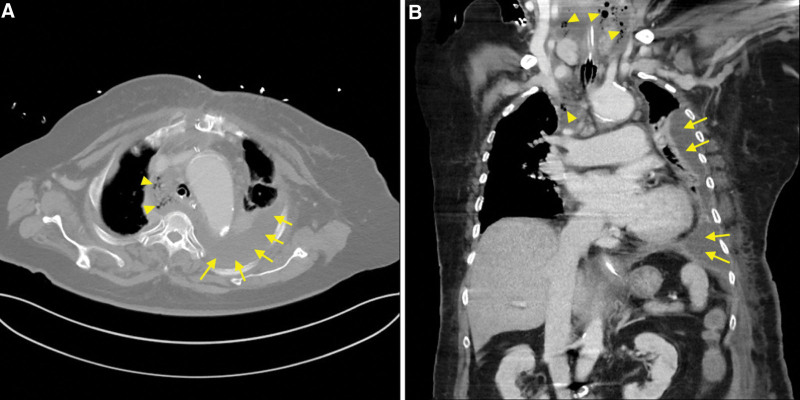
Computed tomography scans of the chest taken 6 d after detection of subcutaneous emphysema confirmed the development of empyema and mediastinal abscess. (A) Axial view and (B) Sagittal view. Arrows indicate pus collects in the left pleural cavity and mediastinum. Arrowheads indicate air locules in the subcutaneous and mediastinal regions.
